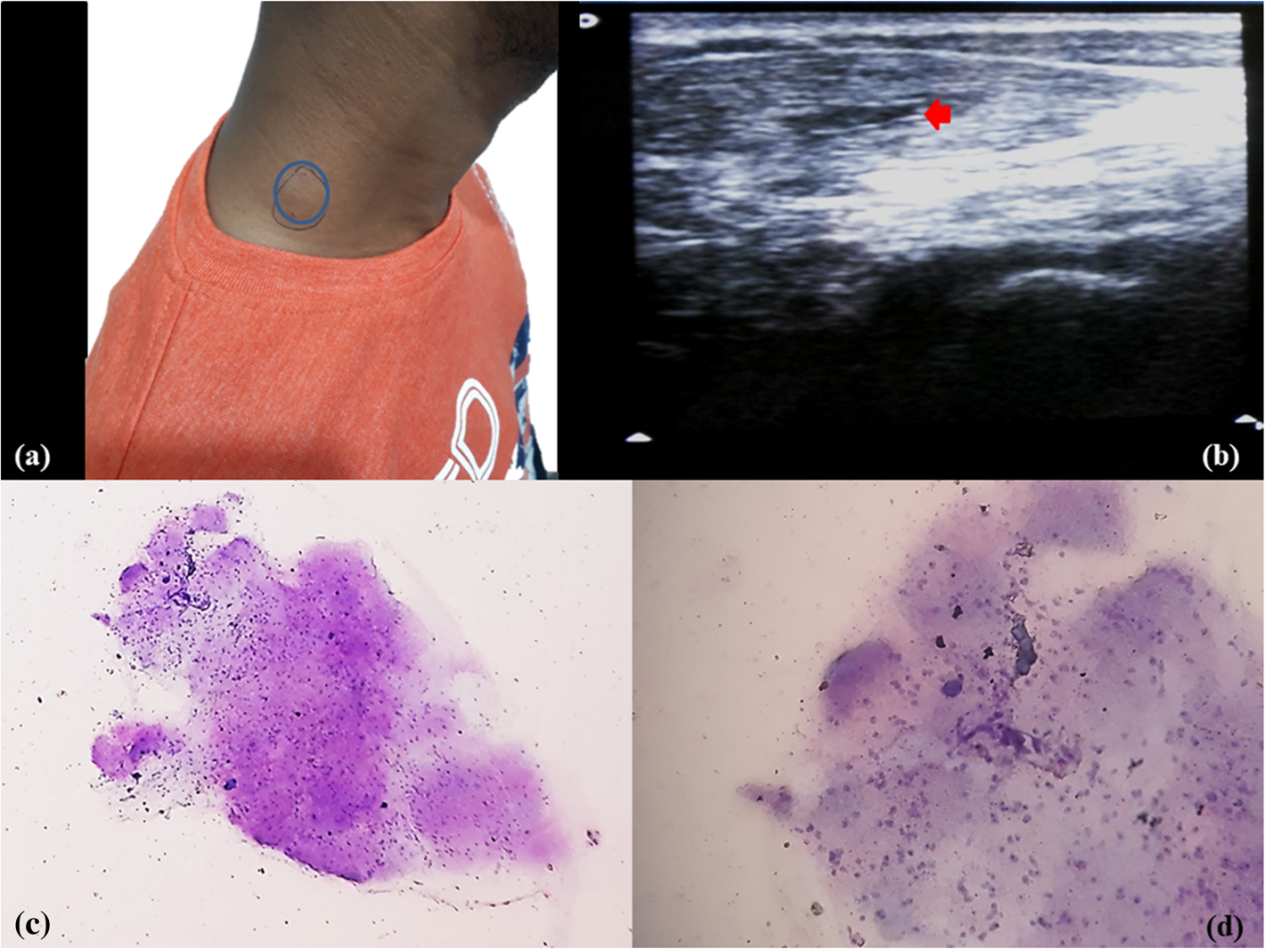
Case 2. a, b Swelling in right neck region; ultrasonography of the neck showed a 2 × 1.5 cm irregular cystic lesion in right scalene muscle. c, d Cytology showed granular bladder wall with subcuticular cells along with small pyknotic-looking nuclei and fibrillary parenchyma and inflammatory cells. (Toluidine blue, ×10 & x40)
